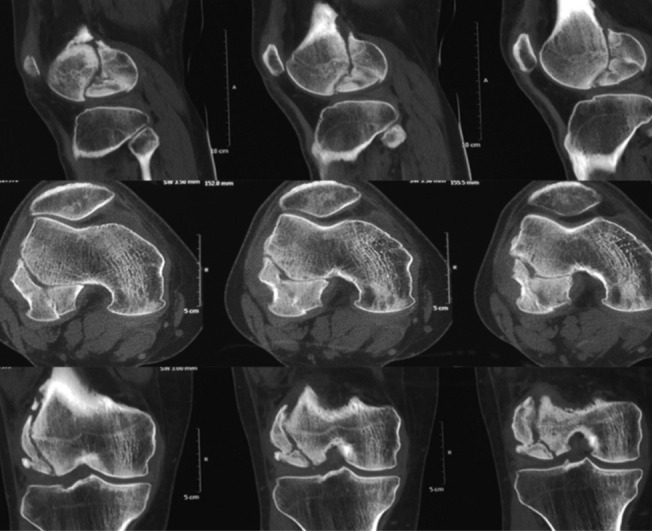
Pre-operative CT scan showing Hoffa fracture of lateral condyle.
